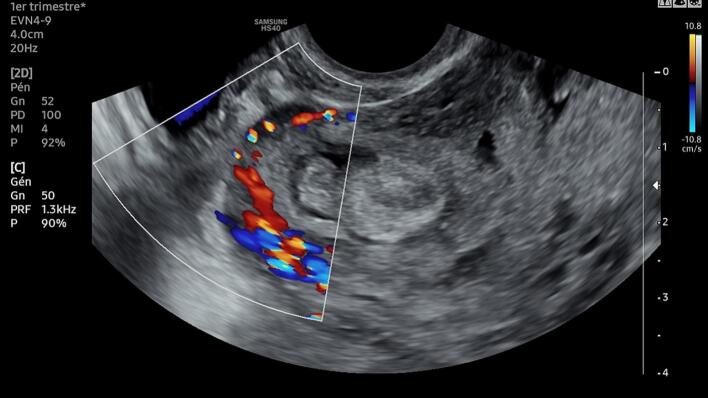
Endovaginal ultrasound showing a low implanted pregnancy with a thin uterine-placental interface, and a turbulent blood flow.
